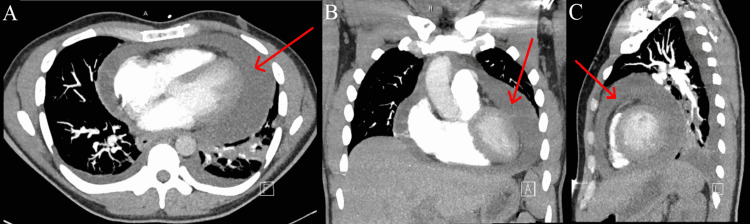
(A) CT PE protocol transverse section of thorax on September 2021. Red arrow marks large pericardial effusion. (B) CT PE protocol coronal section of thorax on September 2021. Red arrow marks large pericardial effusion. (C) CT PE protocol sagittal section of thorax on September 2021. Red arrow marks large pericardial effusion.
